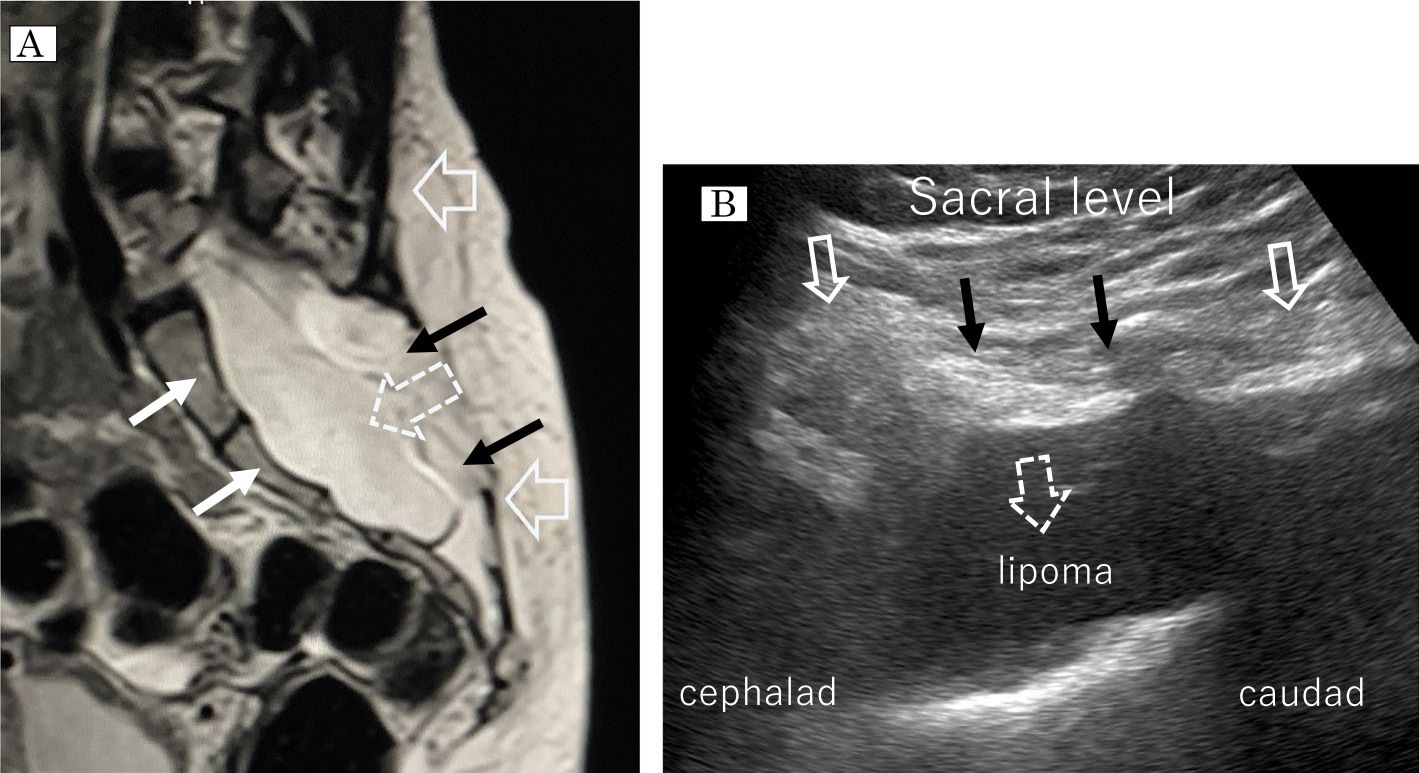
A T2- weighted -magnetic resonance image of sagittal view from the lower lumbar spine to the sacrum. Solid arrows show the defect of the normal bony structures from the L5 level to the sacrum. The dashed arrow shows a spinal lipoma at the level of the sacrum. Two black arrows show the soft tissue continuity from the skin to the lipoma. Two white arrows show the segmented frontal sacrum. B Ultrasonographic image of sagittal view from the lower lumbar spine to the sacrum. Solid arrows show the defect of the normal bony structures of the sacrum. The dashed arrow shows a spinal lipoma at the level of the sacrum. Two black arrows show the soft tissue continuity from the skin to the lipoma
